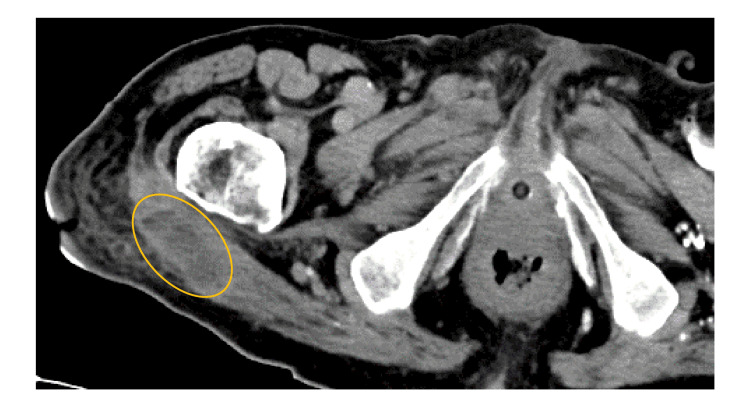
CT findings Defined fluid with a non-enhancing wall (yellow circle) in the swollen right gluteus maximus on contrast-enhanced CT images. CT: computed tomographic
